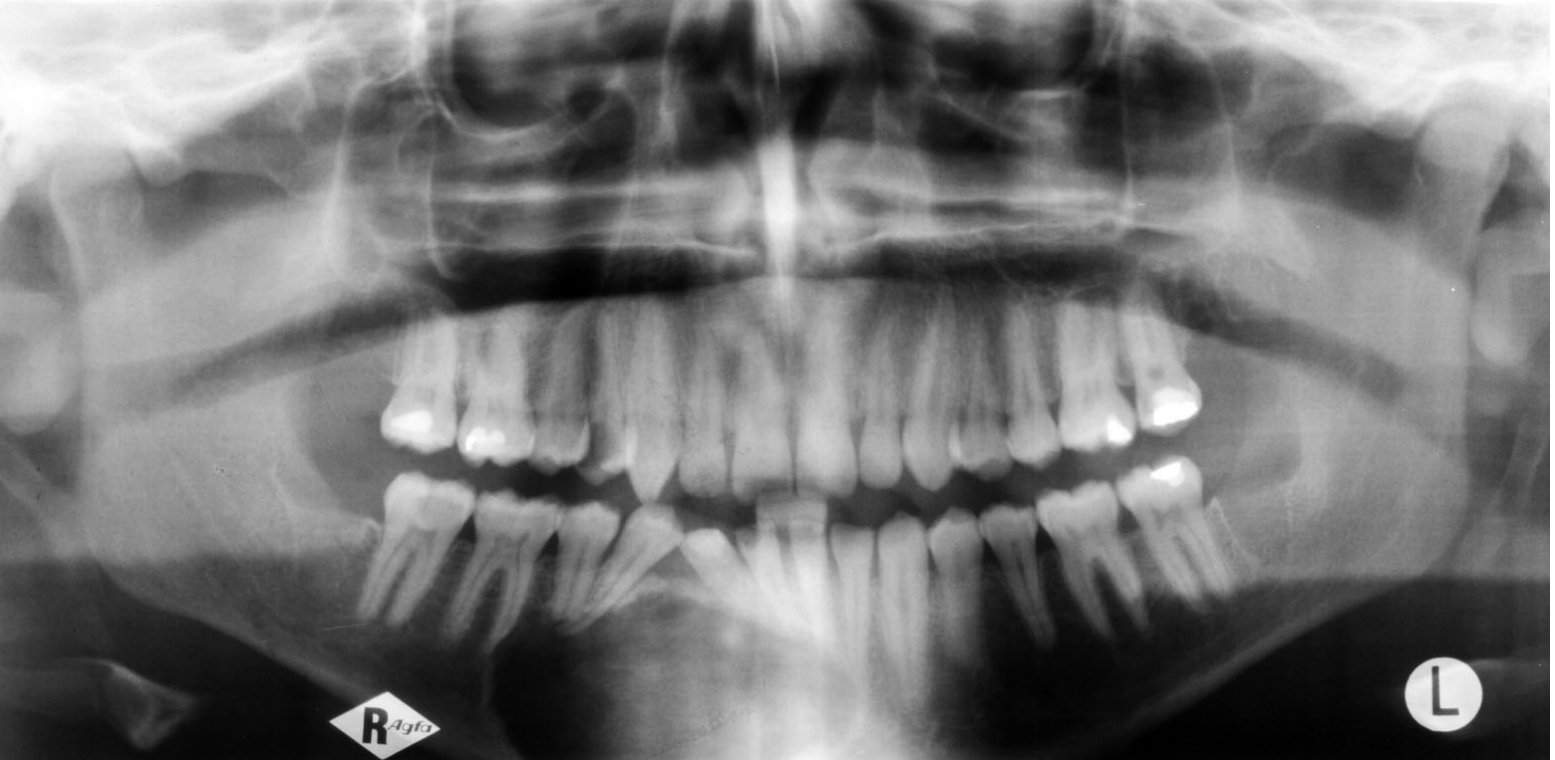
Panoramic radiograph six months after therapy. No root resorption could be observed.
